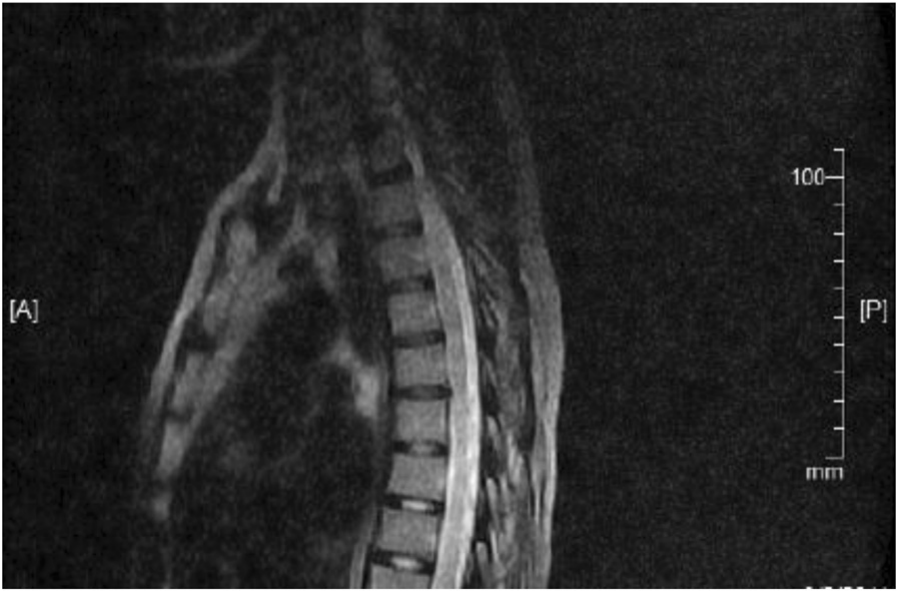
Magnetic resonance imaging studies. Sagittal T2-weighted magnetic resonance imaging showed hyperintense ill-defined lesions from T4 – T8.
